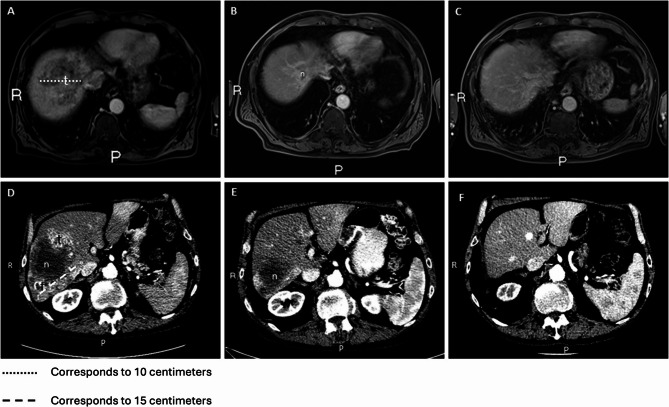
Contrast-enhanced magnetic resonance (MR) images of an 82-year-old patient with advanced HCC (a) at diagnosis (b) at 6-month follow-up after using cannabis oil (c) at 6 years after diagnosis. Contrast-enhanced computed tomography (CT) images of a 77-year-old patient with advanced HCC (d) at diagnosis (e) at 6-month follow-up after using cannabis oil (f) at almost 4 years follow-up after using cannabis oil. N necrosis, t tumor
